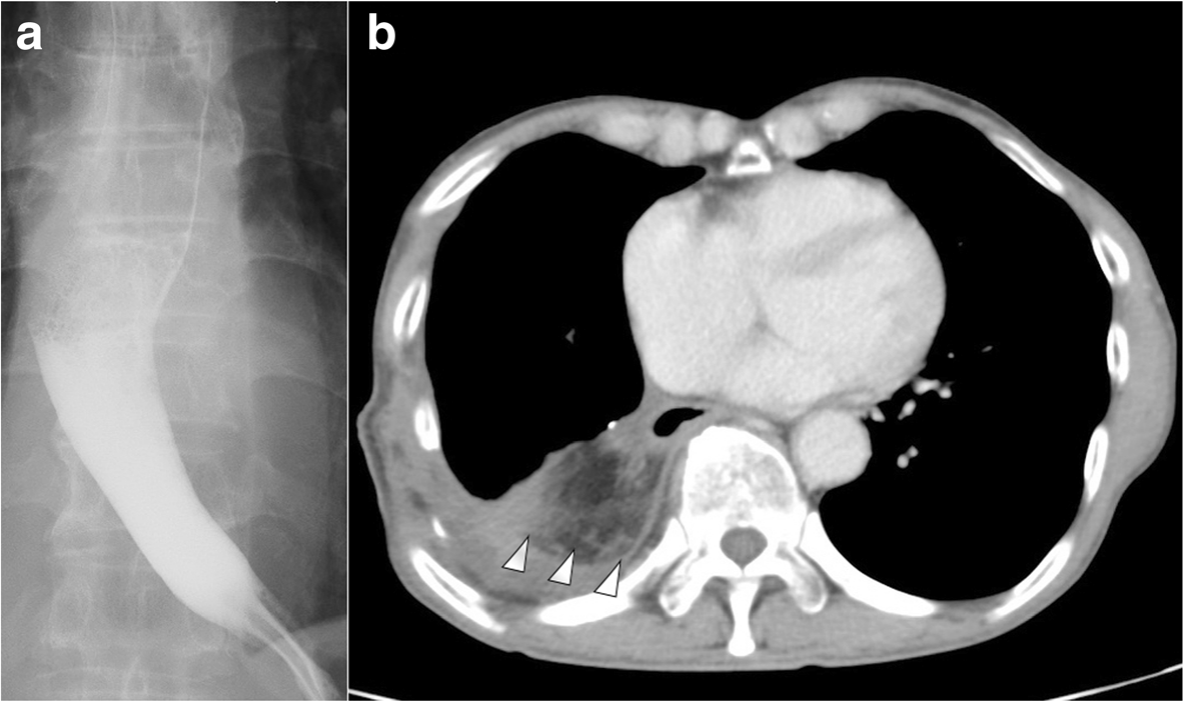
Postoperative image findings. a An esophagogram obtained 7 weeks after the operation showed neither leakage of the contrast medium from the esophageal wall, nor stenosis of the esophagus. b Chest CT performed 5 months after omentopexy showing the omental pedicle flap filling the surplus pleural space in the right thoracic cavity (white arrowheads)
